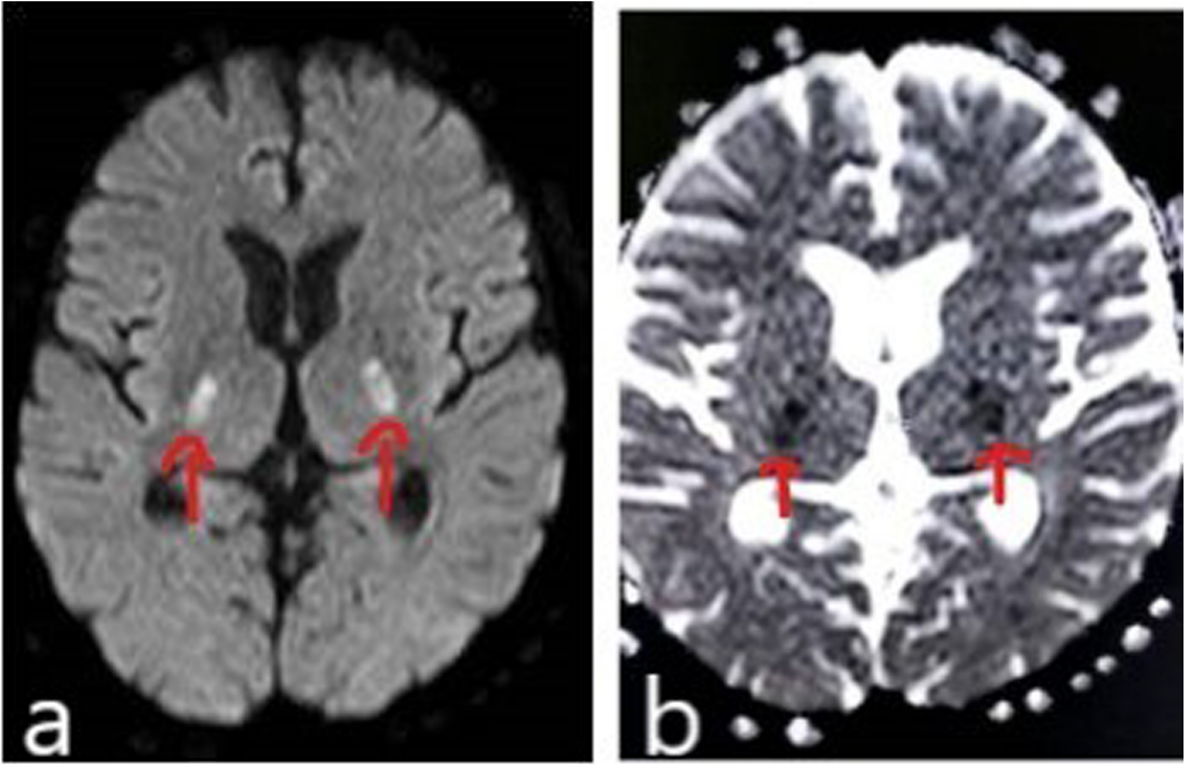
DWI (a) and corresponding ADC (b) images show restricted diffusion in posterior limbs of bilateral internal capsule (red arrows)
